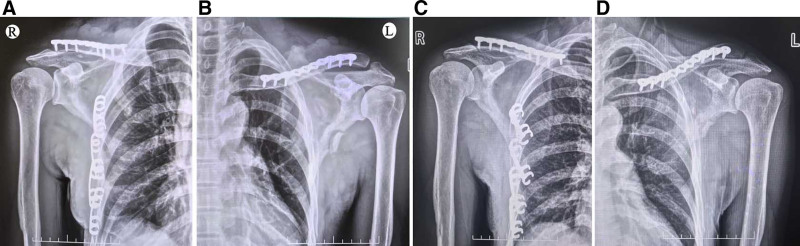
The anteroposterior X-rays of bilateral shoulder joints immediately after the operation and 2 months later.
